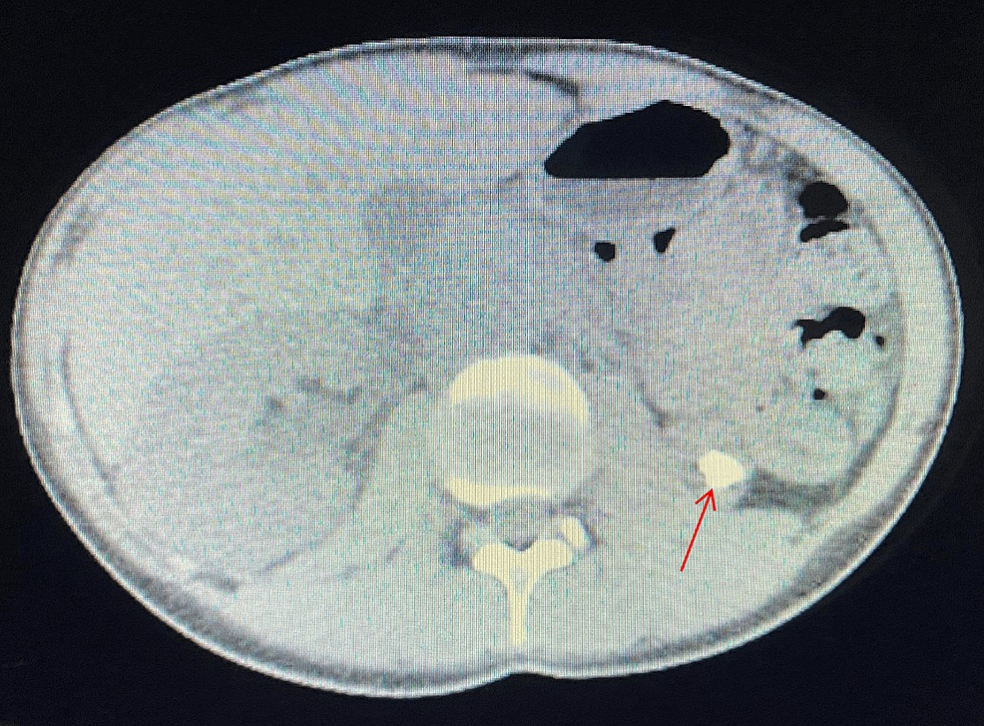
CT showing urinary stone in lower calyx of the left kidney
